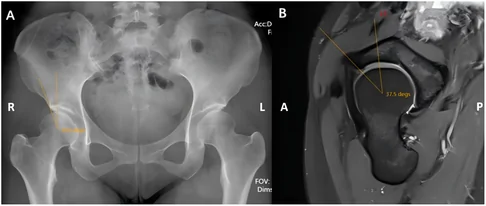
Preoperative radiographic angle measurements of the right hip. (A) Weight‐bearing radiograph measuring the lateral CE angle at 18.6°; (B) Magnetic resonance imaging sagittal view measuring the anterior CE angle at 37.5°. (A, anterior; CE, center‐edge; L, left; P, posterior; R, right.)
